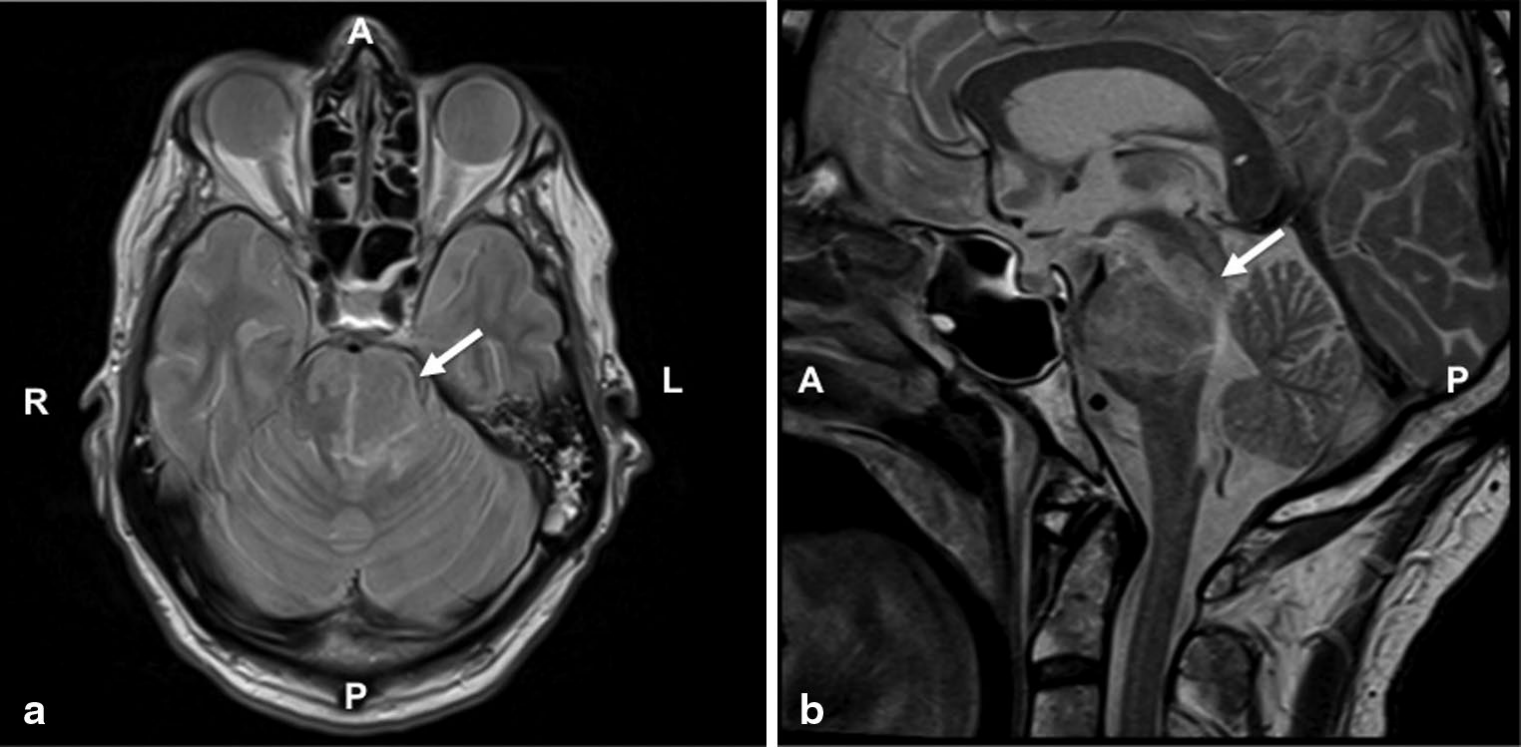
Transversal and sagittal views of the brain stem using T2-weighted magnetic resonance imaging. A left-sided brain stem infarction expands to the cerebral and cerebellar peduncle (*arrows*). *A* anterior, *P* posterior, *R* right, *L* left
